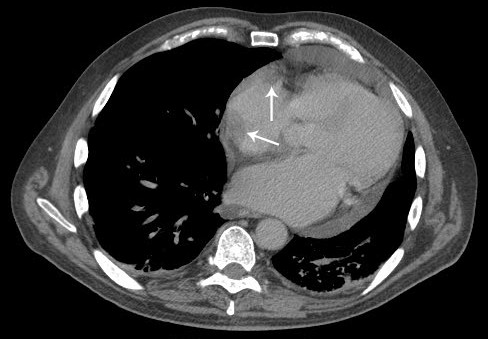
Contrast-enhanced computed tomographic axial image demonstrating filling defects (arrows) corresponding to echocardiographic findings.
